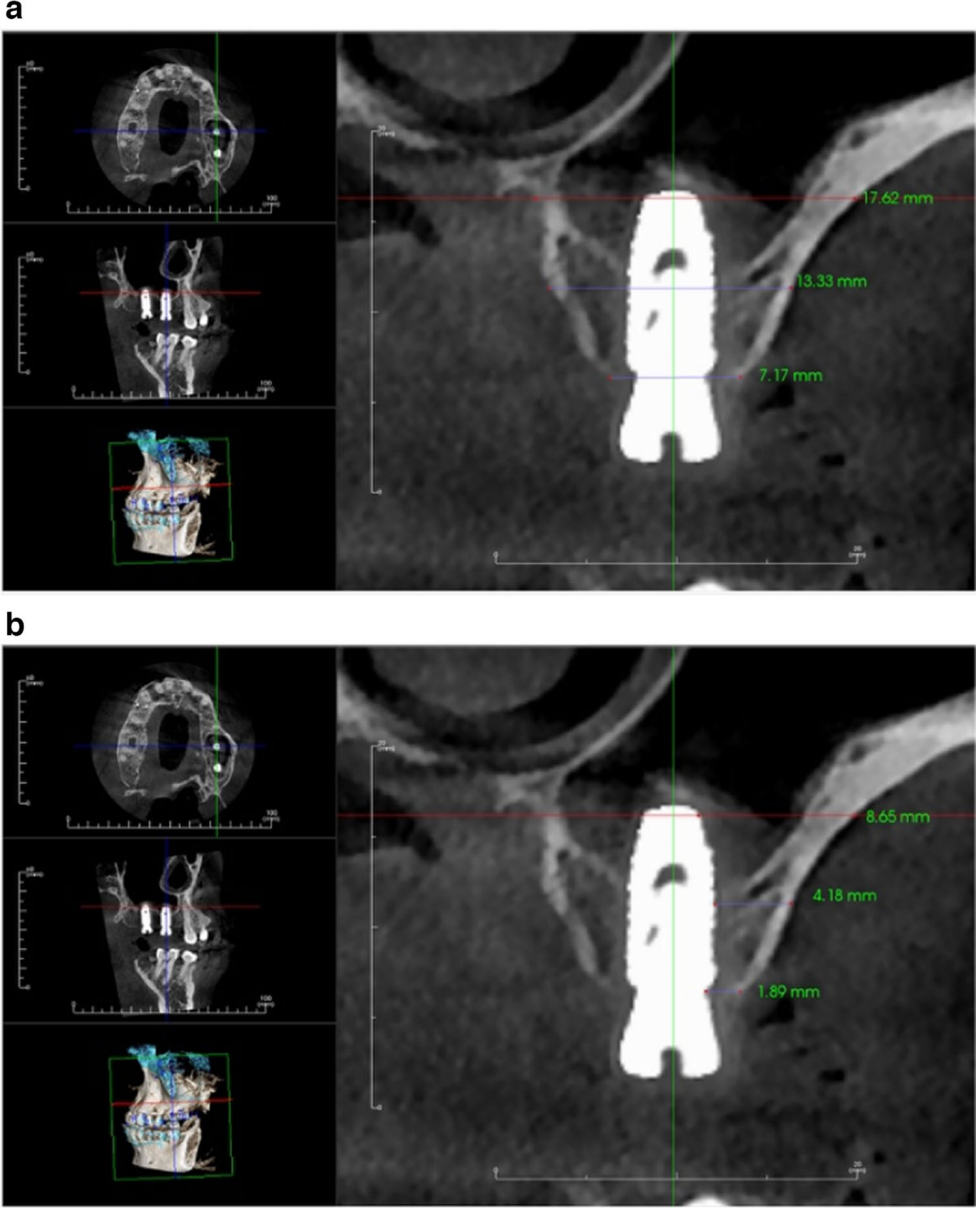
a Immediate postoperative CBCT showing bone width. b Immediate postoperative CBCT showing labial plate of bone thickness
